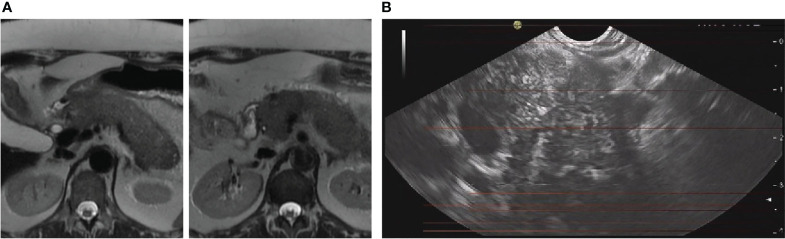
Single-shot fast spin-echo MRI sequence showing diffuse inflammatory infiltration of the pancreas (A). Endosonographic image showing massive hypoechoic swelling, multiple interlobular septa, and diameter changes (B).
